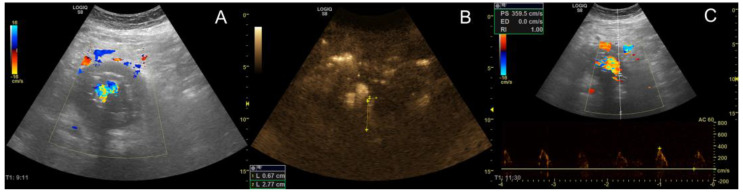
Ultrasound surveillance investigation after EVAR. While the lumbar type II endoleak could not be displayed via Duplex ultrasound (A), B-Flow allowed sufficient visualization (B) that could be validated with pulsed-wave Doppler (C).
